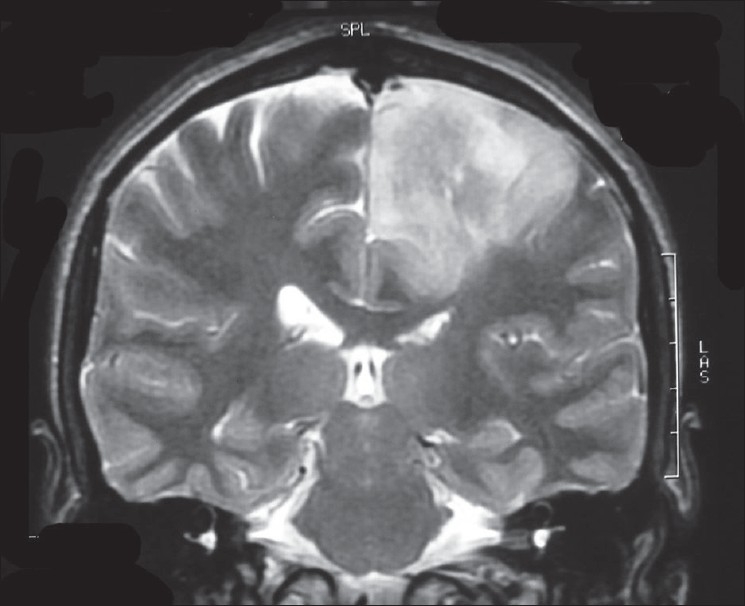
T2 weighted MRI brain coronal section showing parasagittal hyper intense mass with mass effect
